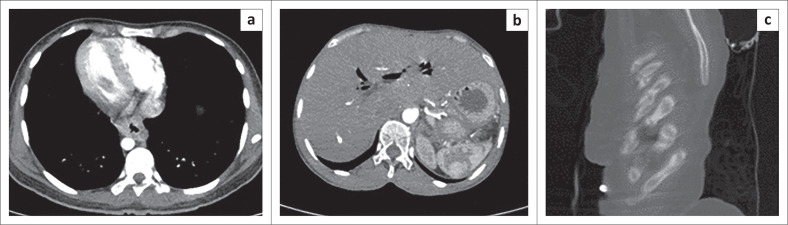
Axial (a, b) and sagittal (c) CT images of incidental extra-pulmonary findings in three pulmonary embolism-negative patients. (a) A patient with circumferential lower oesophageal wall thickening, (b) extensive pneumobilia in a post-surgical patient and (c) patient with multiple chronic rib fractures.
